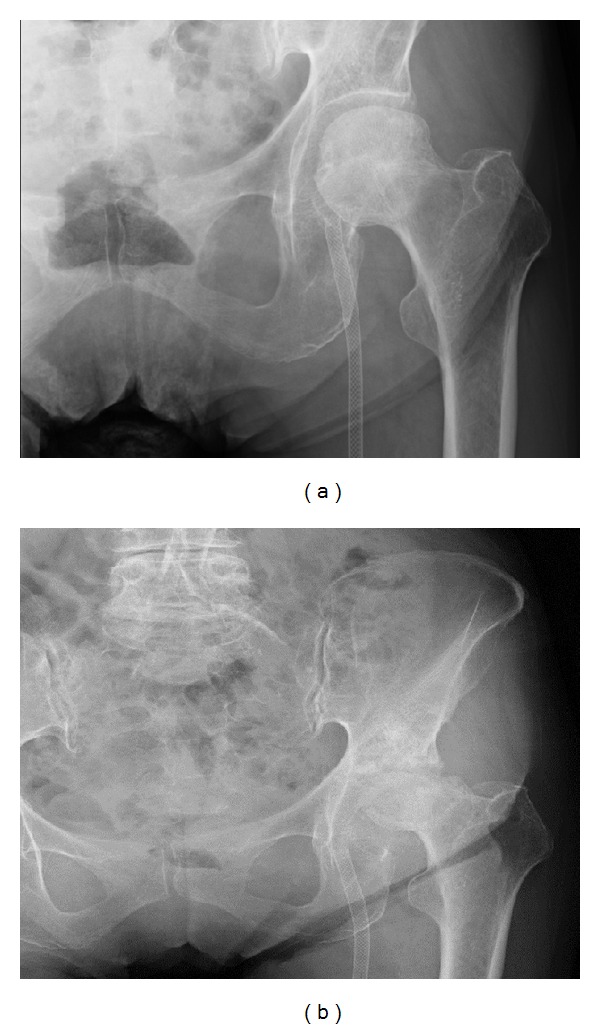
Plain radiography. (a) Just after the stent placement in another hospital. Stent is detained in left femoral artery. Left femoral head was not collapsed. (b) At the time of admission to our hospital. Left femoral head was collapsed and joint space disappeared.
